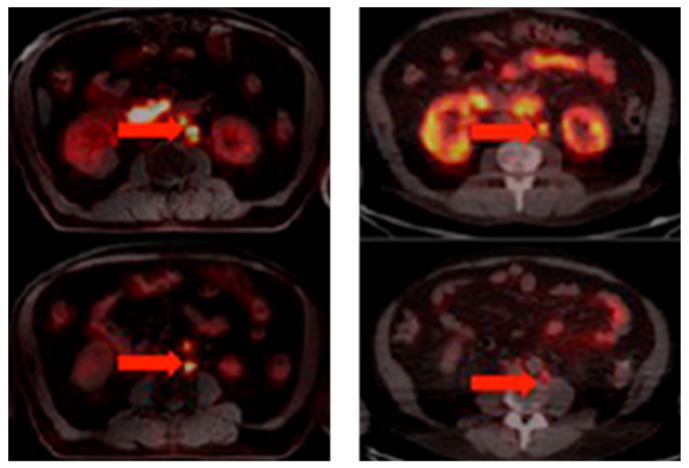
[^68^Ga]Ga-RM2 (left) vs. [^68^Ga]Ga-PSMA-11 (right) PET/CT images of PCa lesions (red arrows) in a patient with LNM. This research was originally published in the Journal of Nuclear Medicine (JNM) [122].
